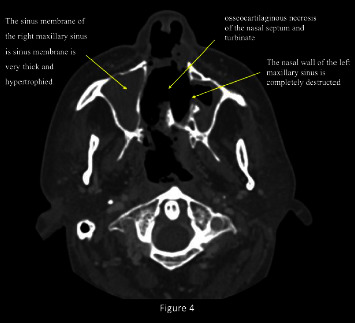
Axial CT scan image showing the necrosis of the osseo-cartilagineous of the nasal septum and turbinate, and the destruction of the nasal wall of the left maxillary sinus and the sinus membrane of the right maxillary membrane is very thick and hypertrophied.
